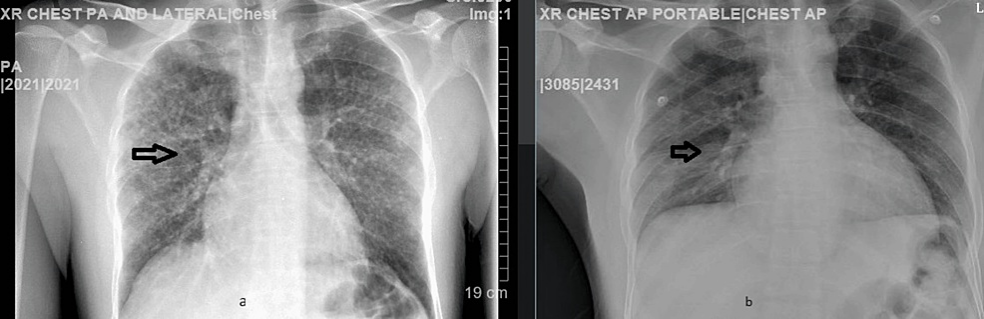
Comparison of CXR during admission and discharge a: On admission, multifocal opacities; b: On discharge, improved multifocal opacities after antimicrobial treatment CXR: Chest X-ray
